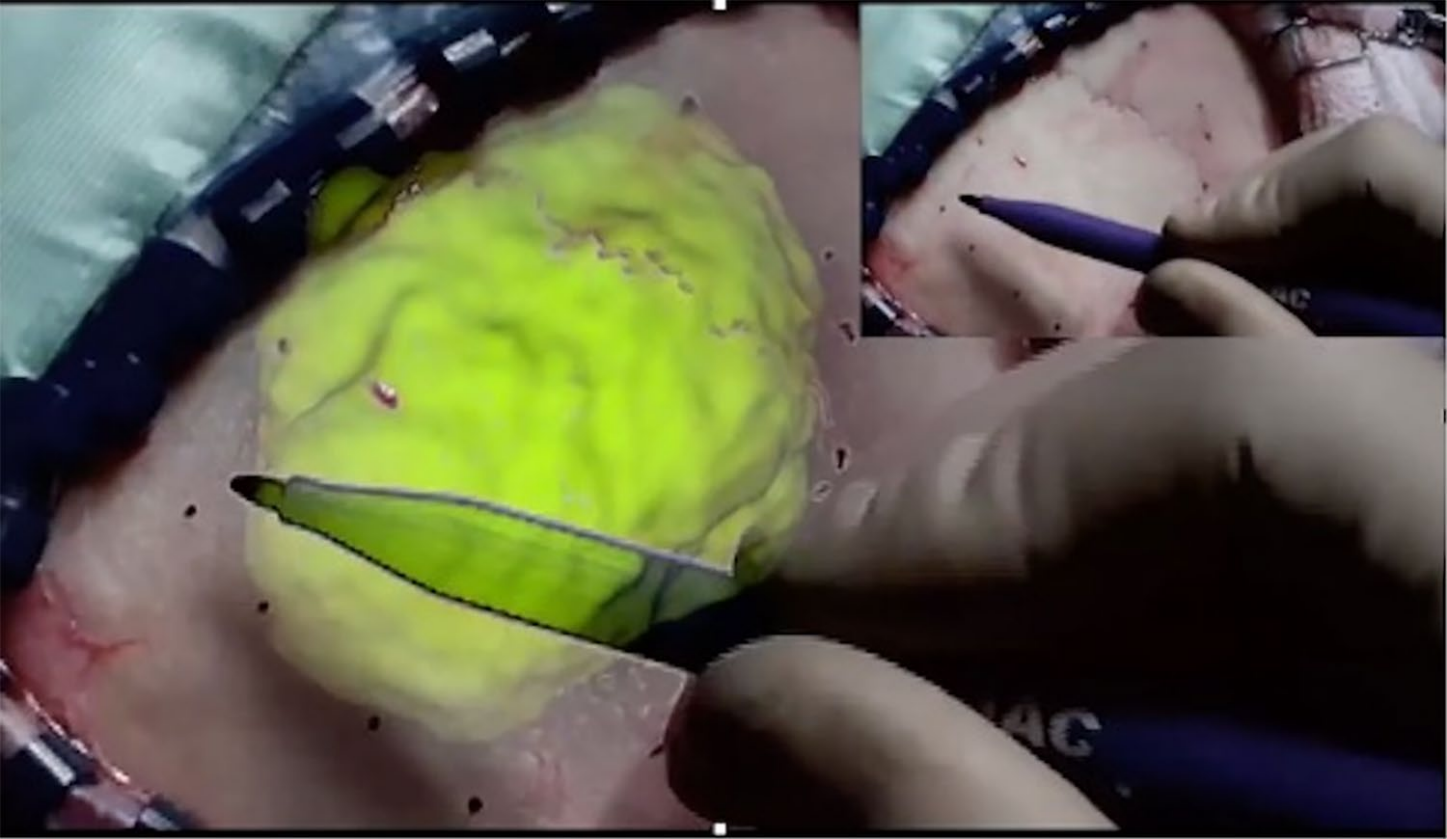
An example of a tumor hologram derived from MRI data and visualized in the HoloLens together with the “real world” scalp, after the anchorage procedure. The mixed-reality approach allows an immediate and clear understanding of the tumor position underneath the skull, helping the surgeon to delineate the best area for craniotomy
